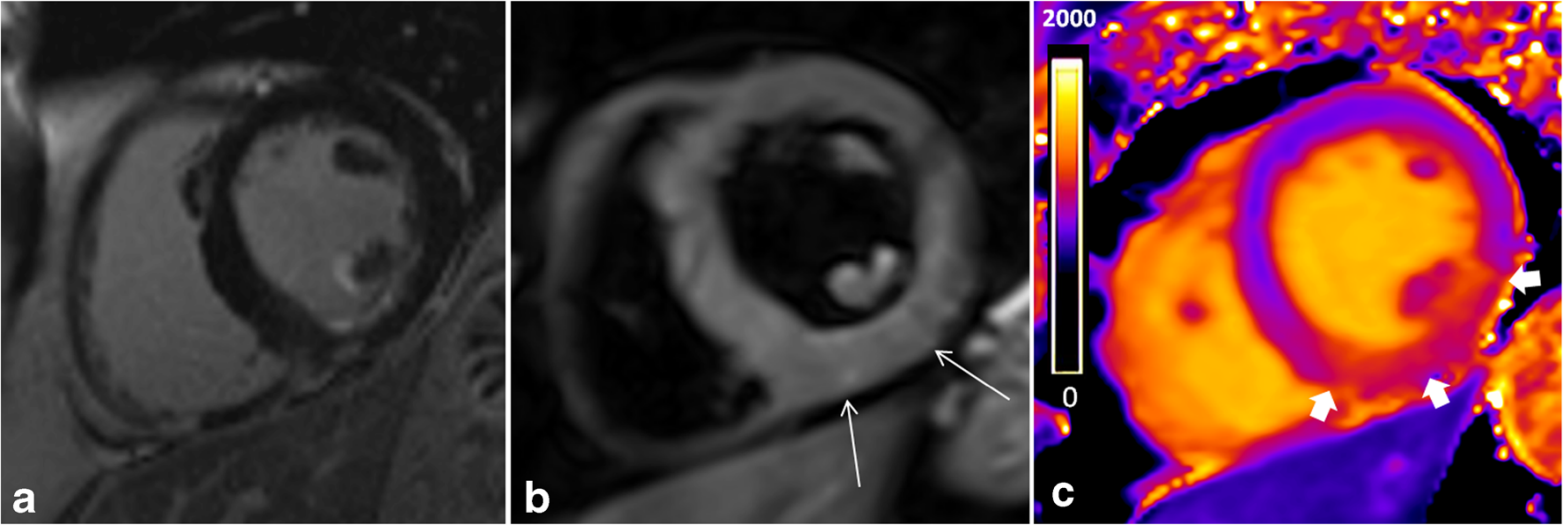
T1 mapping in acute myocardial infarction. Subendocardial enhancement (a) in the inferolateral, midventricular segment of the left ventricle. Although the T2-weighted images (b) show only a mild increase in brightness (*long arrows*), there is an area of increased T1 values (1,208 ms, into the *orange* range of the colour scale) (*short arrows*) (c) exceeding the area of LGE enhancement
